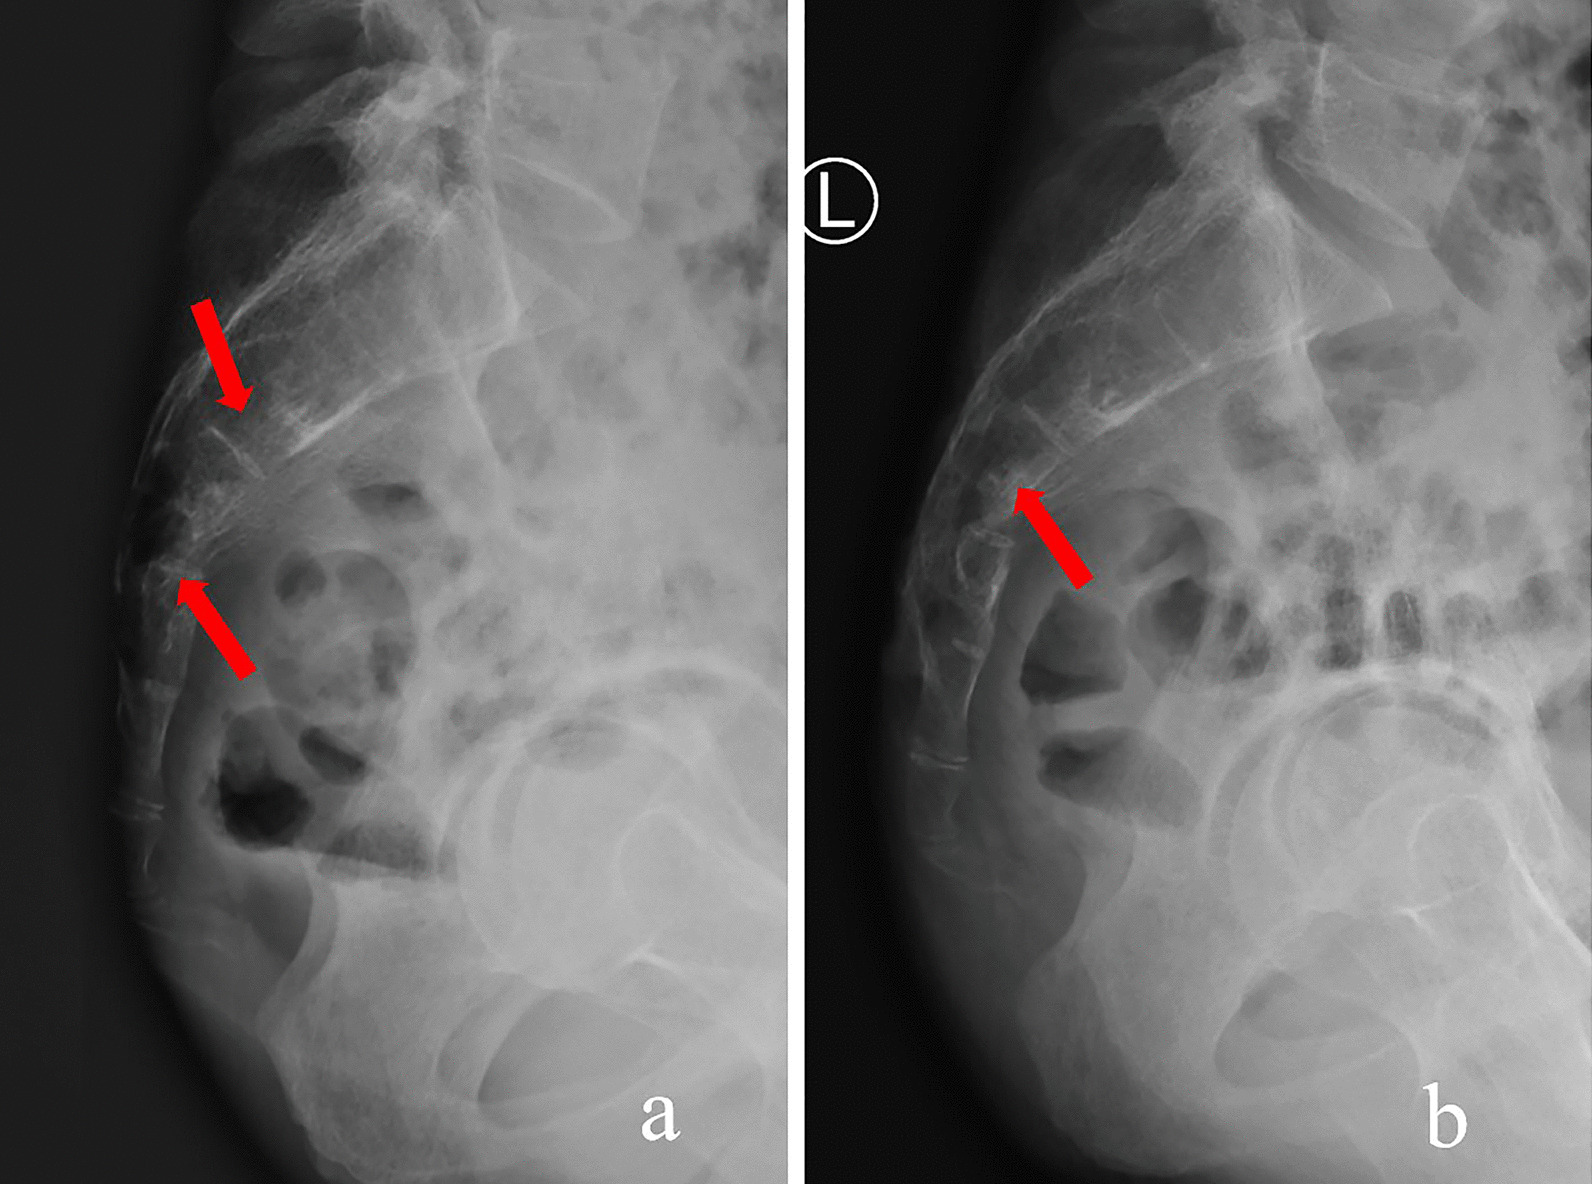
Lateral radiography (sacrum). a Preoperative radiograph: patchy osteolytic lesions in S2–S4 with an unclear boundary (red arrows). b Radiograph, 6-month postoperatively: bone defect lesions of the sacrum with clear boundary and rim sclerosis (red arrows)
